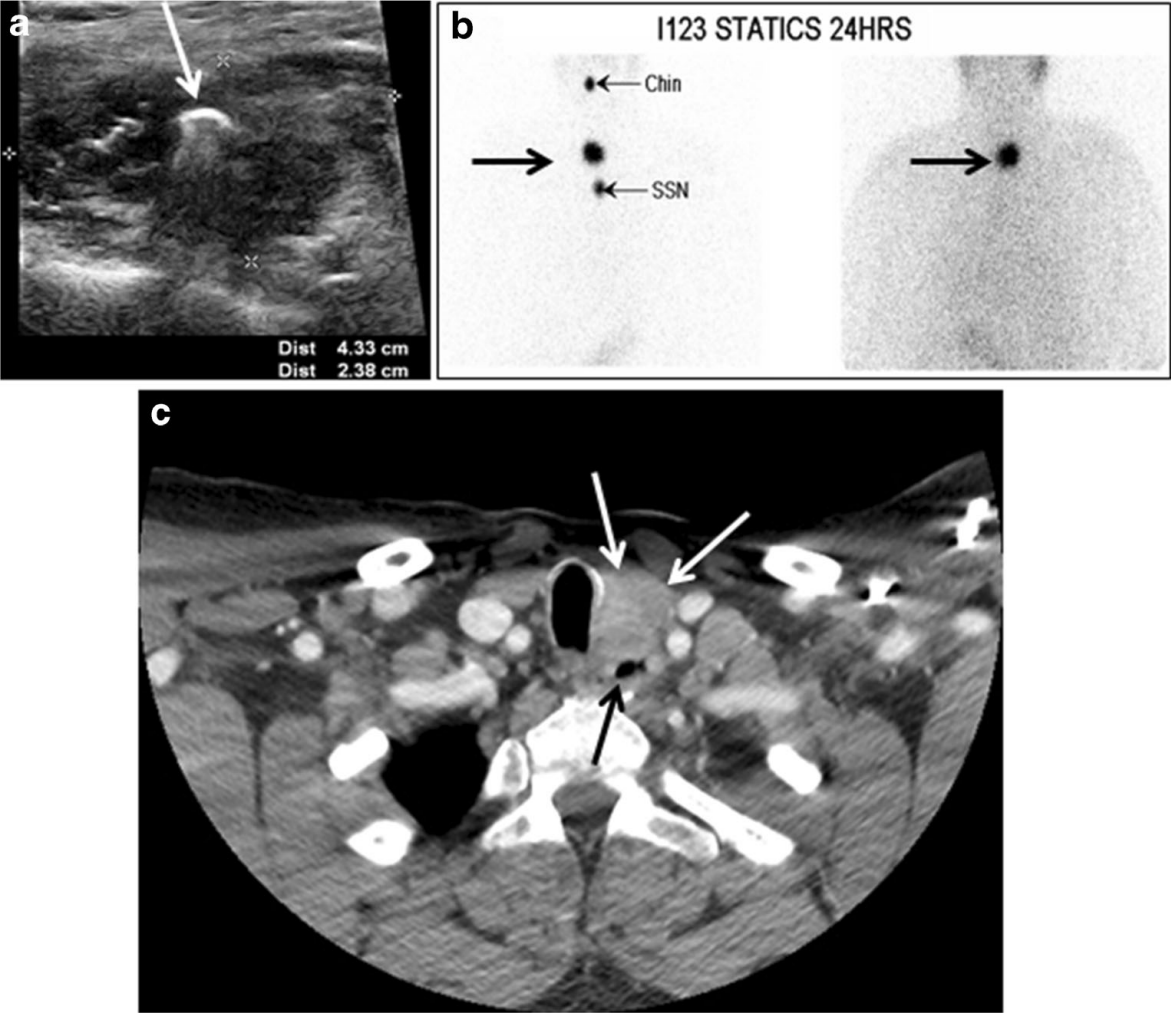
A 48-year-old male patient post total thyroidectomy with PTC recurrence. a Transverse greyscale ultrasound of the neck demonstrates a left thyroid bed heterogeneous, predominantly hypoechoic irregular lesion with calcifications (*white arrow*). b A spot image of iodine 123 total body scan of the neck demonstrate a focus of abnormal radiotracer uptake at the left thyroid bed (*Black arrows*) between the annotated markers. c Enhanced axial CT scan of the neck demonstrates an enhancing large left thyroid bed mass (*white arrow*) with no calcifications. The lesion exerts a mass effect on the oesophagus (*black arrow*) and is inseparable from the trachea
